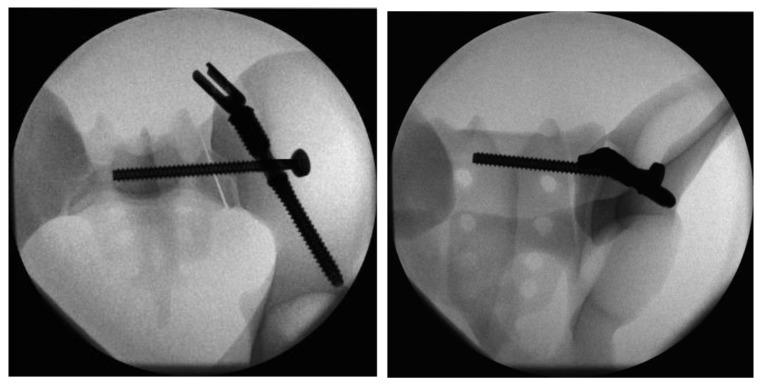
Radiographs in two planes of the triangular fixation system with the sacroiliac screw positioned through the fenestra of the iliac screw.
